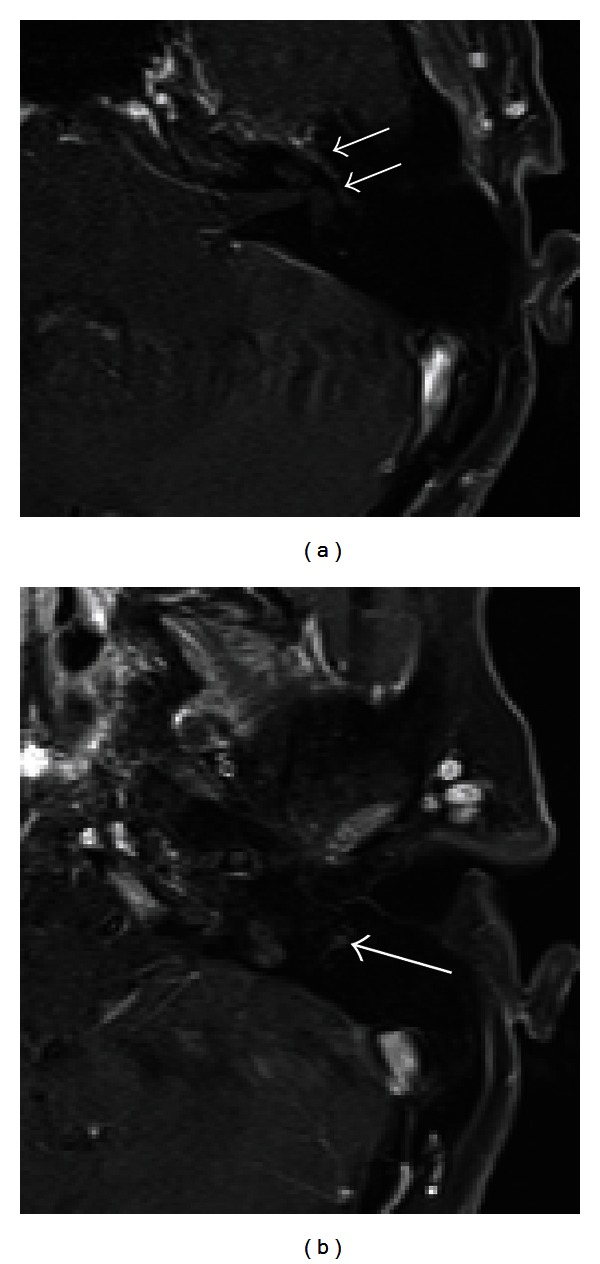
Normal facial nerve on MRI. Postcontrast fat-saturated T1-weighted MRI images of normal enhancing tympanic (a) and mastoid (b) CN VII segments.
